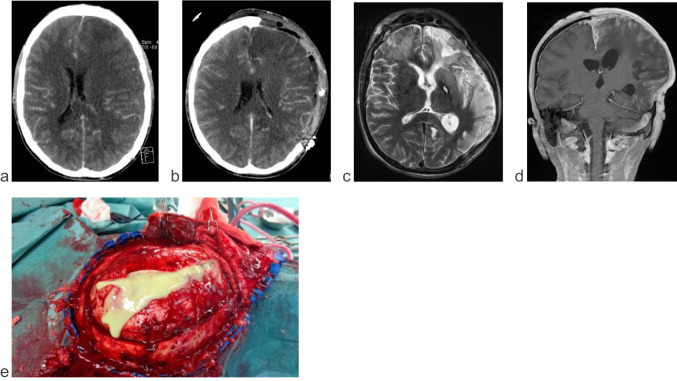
Patient with intracranial space-occupying left hemispheric and interhemispheric subdural empyema. a Contrast-enhanced axial scan CT at admission. b Follow-up CT scan 7 days after emergency hemicraniectomy and evacuation of left hemispheric empyema. c T2-weighted axial scan MRT and d T2-weighted FLAIR coronar scan, 4 weeks after admission. e Intraoperative situs with emission of pus after opening of the dura
